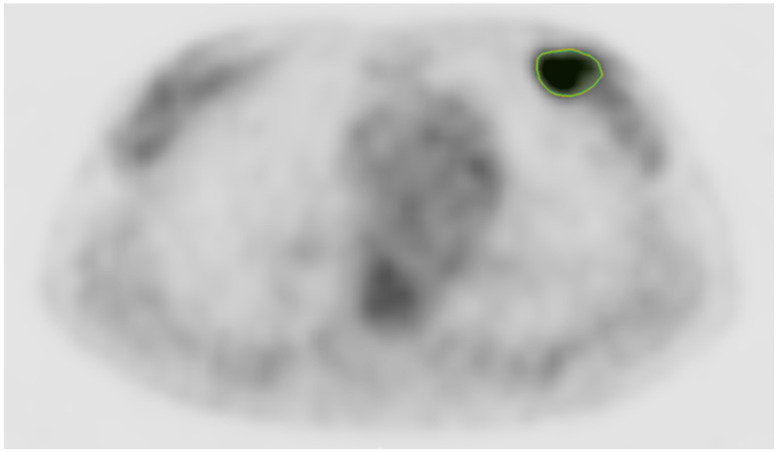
Example of 3D segmentation using a semi-automatic method based on 42% of the SUV_max_. The green contour delineates the segmented primary breast tumor lesion.
